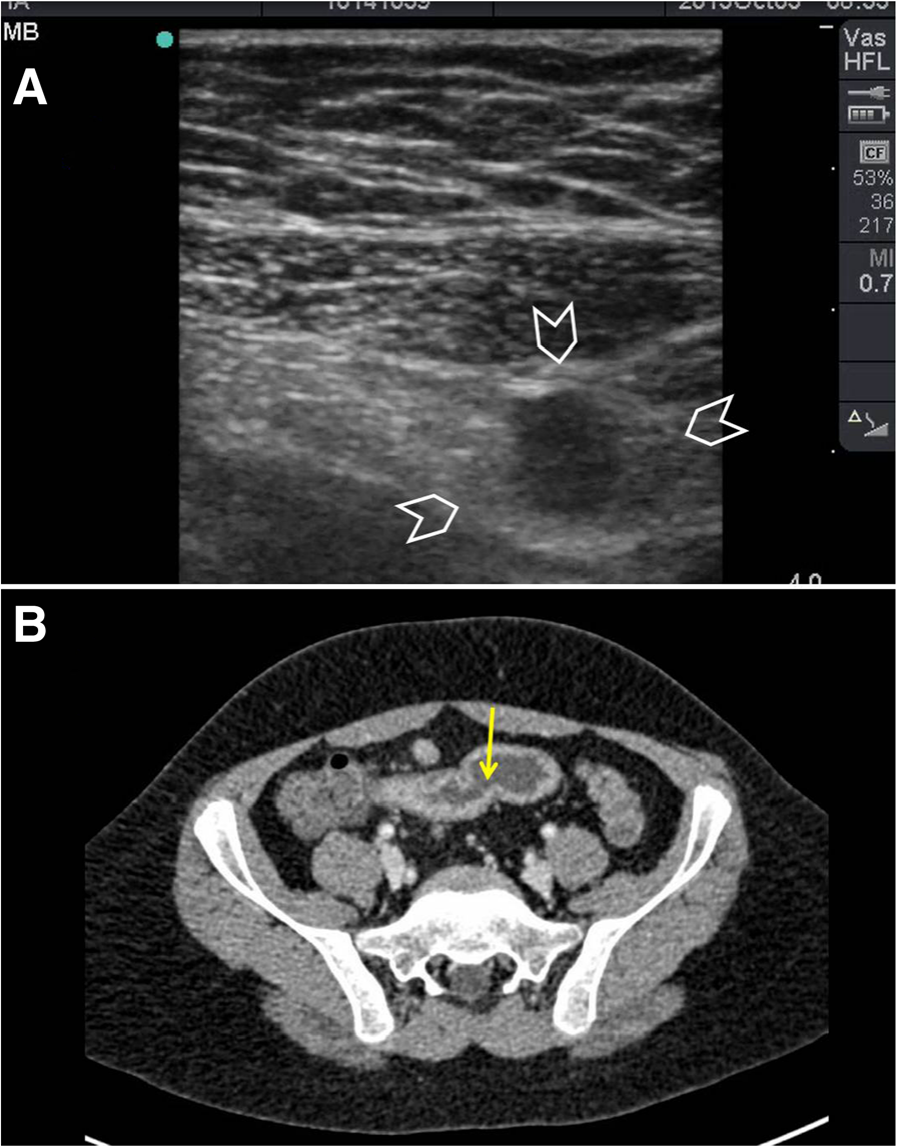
A 14-year-old boy presented with pain in the right iliac fossa of 1 month duration associated with diarrhoea. His abdomen was soft and tender at the right iliac fossa. POCUS (a) using a high-frequency linear probe of the right iliac fossa showed a thickened hyperechoic non-compressible small bowel (arrowheads). The bowel layers could not be seen. Crohn’s disease was suspected. CT with intravenous contrast (b) showed a thickened constricted terminal ileum suggestive of Crohn's disease (yellow arrow)
